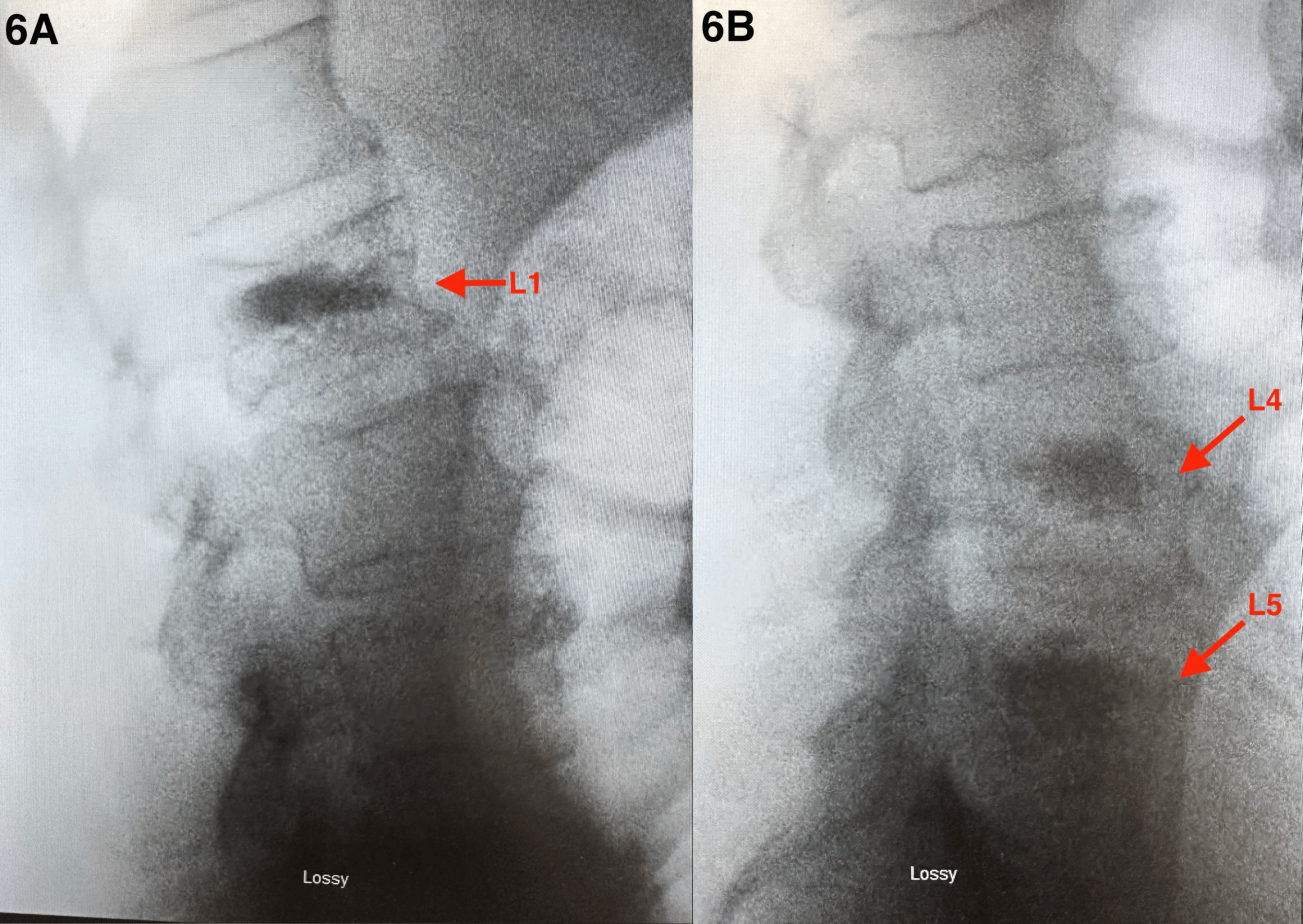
Postoperative lateral lumbar spine radiographs demonstrate vertebral body augmentation at L1 (A), L4, and L5 (B) with increased radiodensity consistent with kyphoplasty cement placement. Restoration of vertebral body height is noted without evidence of cement extravasation, posterior wall retropulsion, or spinal canal compromise. Findings are consistent with post-procedural stabilization of vertebral compression fractures. Image captured using a smartphone camera due to limitations in accessing downloadable imaging files. All identifiable information was removed.
